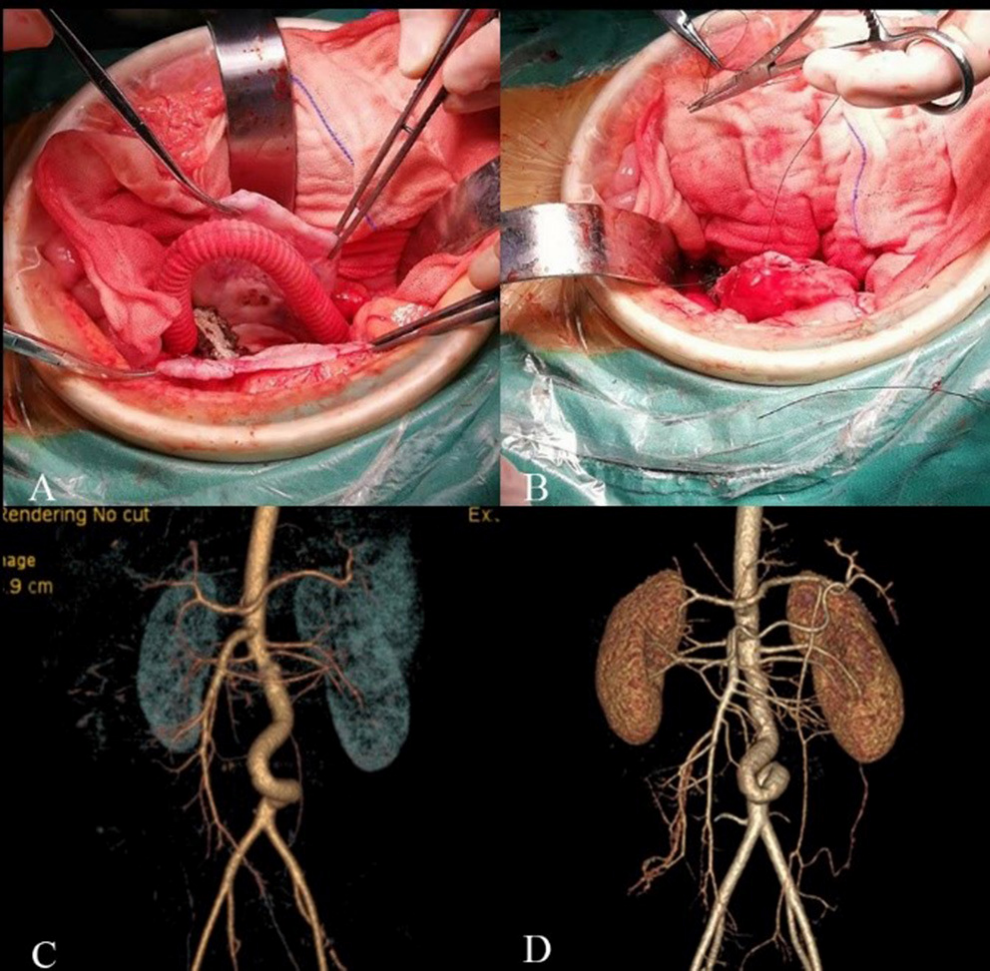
Intraoperative and follow-up images. (A) A 10 mm Dacron aorto-aortic tube graft replace the AAA with a “C” shape. (B) The aneurysmal sac was wrapped around the graft. (C) CTA 7 days after surgery. (D) CTA 40 months after surgery showed twisted but unobstructed graft.
